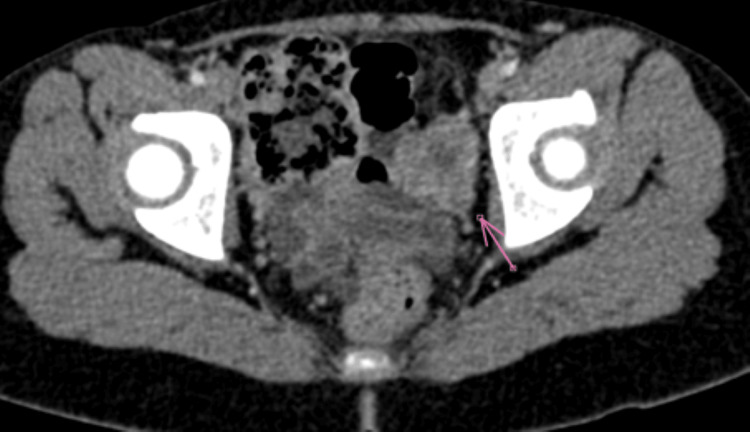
Abdominopelvic CT showing a suspicious left lateral uterine mass (arrow) of likely ovarian origin.
